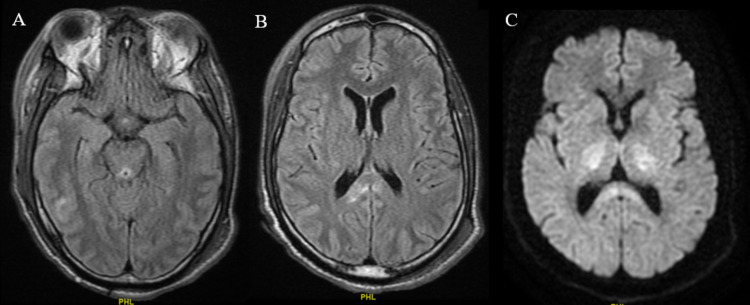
Magnetic resonance imaging (MRI) brain. A. T2-weighted Fluid-Attenuated inversion recovery (T2 FLAIR) image: Increased signal within the periaqueductal gray matter and extending along the dorsal aspect of the brainstem. B. T2 FLAIR image: Hyper-intense signal within the right greater than left splenium of the corpus callosum. C. Diffused-weighted (DWI) image: Subtle restricted diffusion and expansion of the posterior limbs of each internal capsule.
